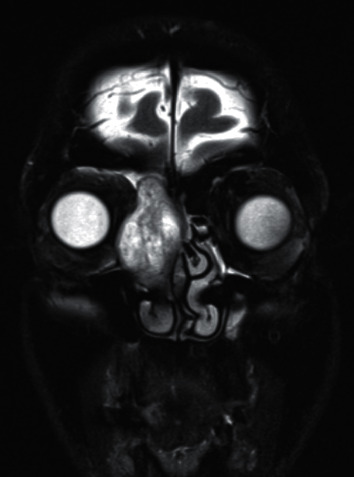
Pre-op MRI scan (coronal plane). Preoperative MRI T2 sequence showing the tumour measuring 40 × 23 mm obstructing the frontal sinus drainage pathway with infraorbital extension and bowing of the nasal septum.
